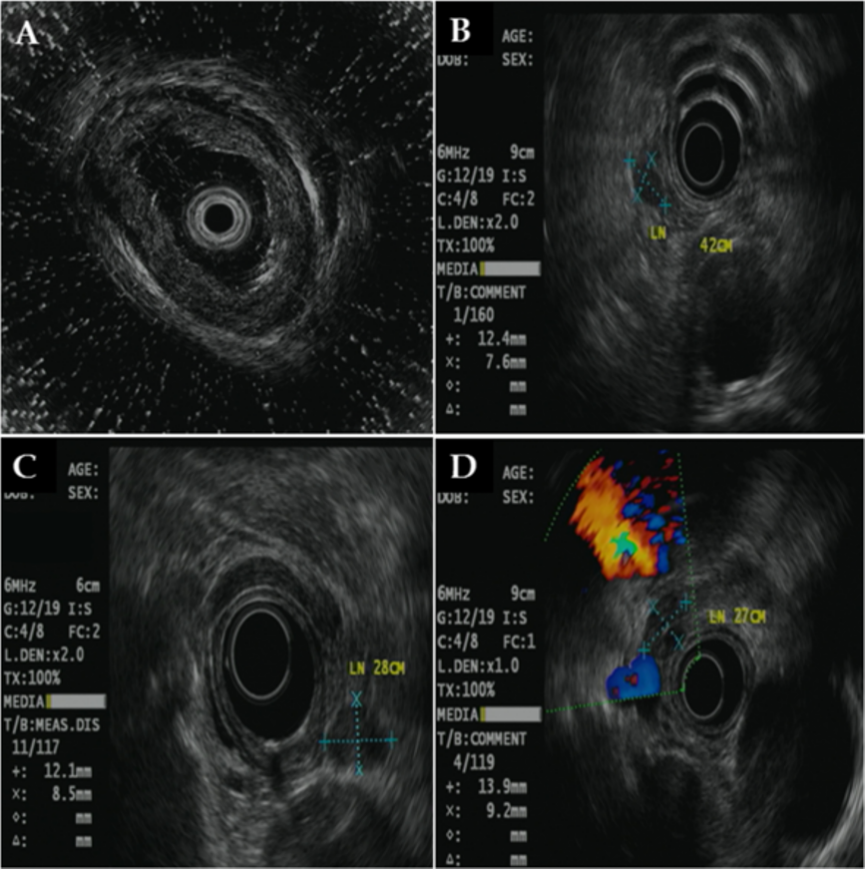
Endoscopic ultrasonography findings. (A) Blurring and thickening of the submucosal layer were observed, but the proper muscle layer was spared. Three enlarged regional lymph nodes (>10mm) were observed at 42cm (B), 28cm (C) and 27cm (D) from the incisor.
